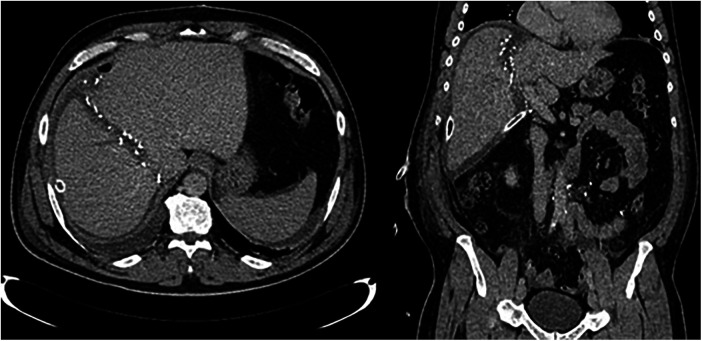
CT images after stage one showing partitioned liver and surgical clips along the transection line.
